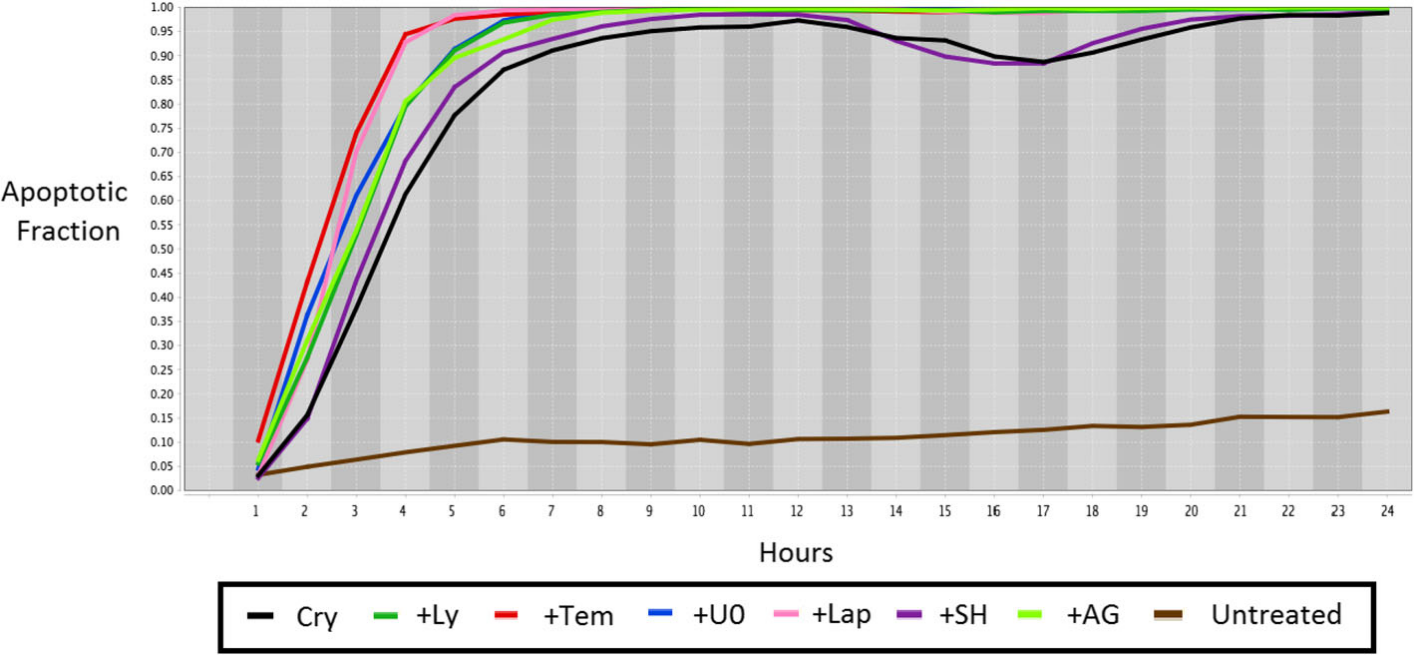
Experimental results for each single drug in combination with CT
